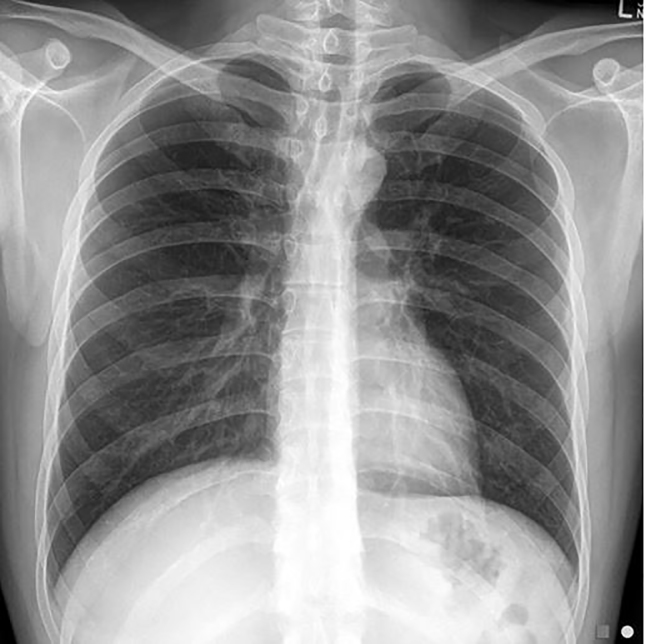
Radiograph of the patient’s chest while in the emergency department showing no acute disease (posterior-anterior view).
